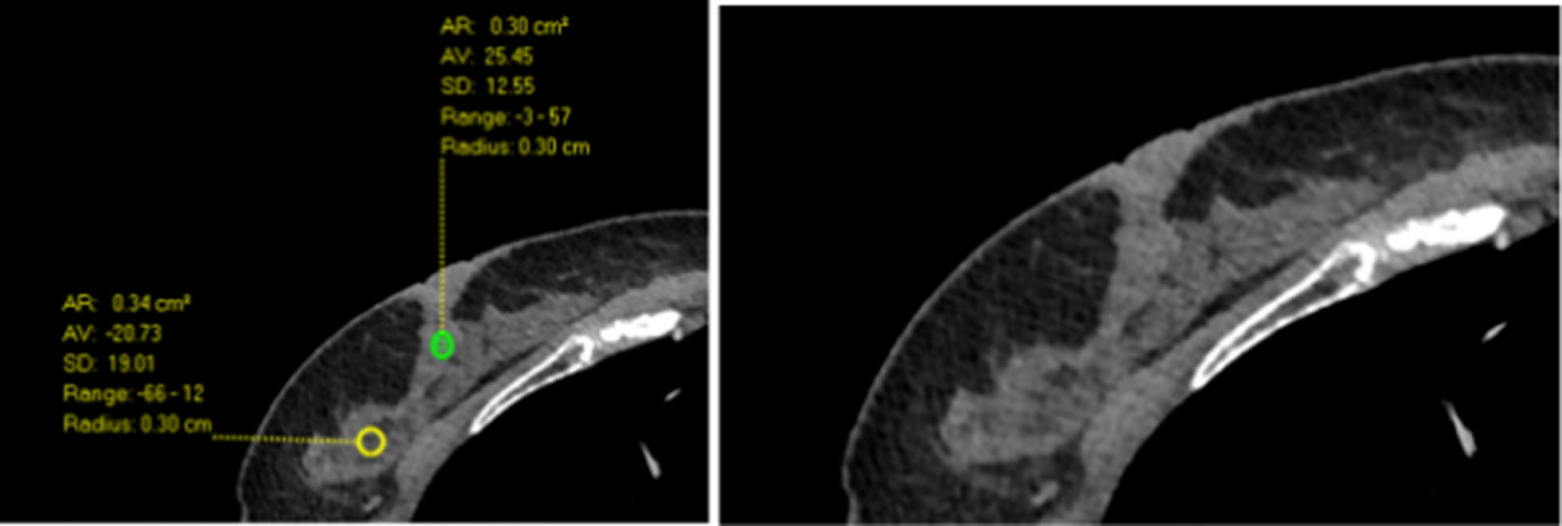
Axial chest CT image measuring 2 regions of interest in the right breast. The yellow circle has a mx HU of 12 and therefore is less dense that the green circle with HU of 57 which is denser
